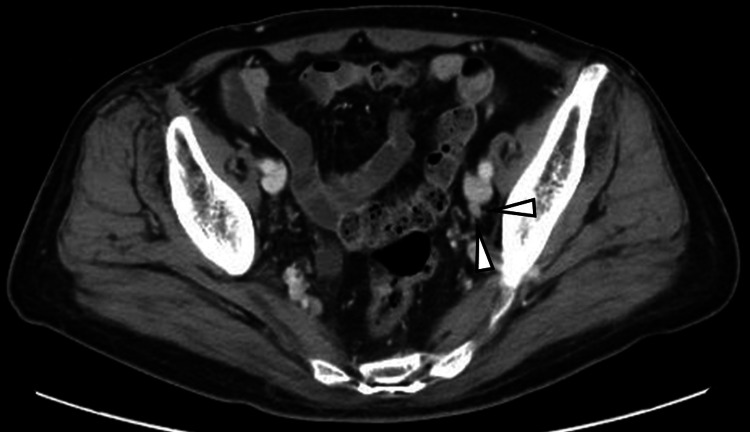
Left external iliac lymph node involvement as a new lesion After two courses with chemotherapy, computed tomography revealed left external iliac lymph node involvement as a new lesion, although the bladder cancer and right external iliac lymph node decreased in size.
